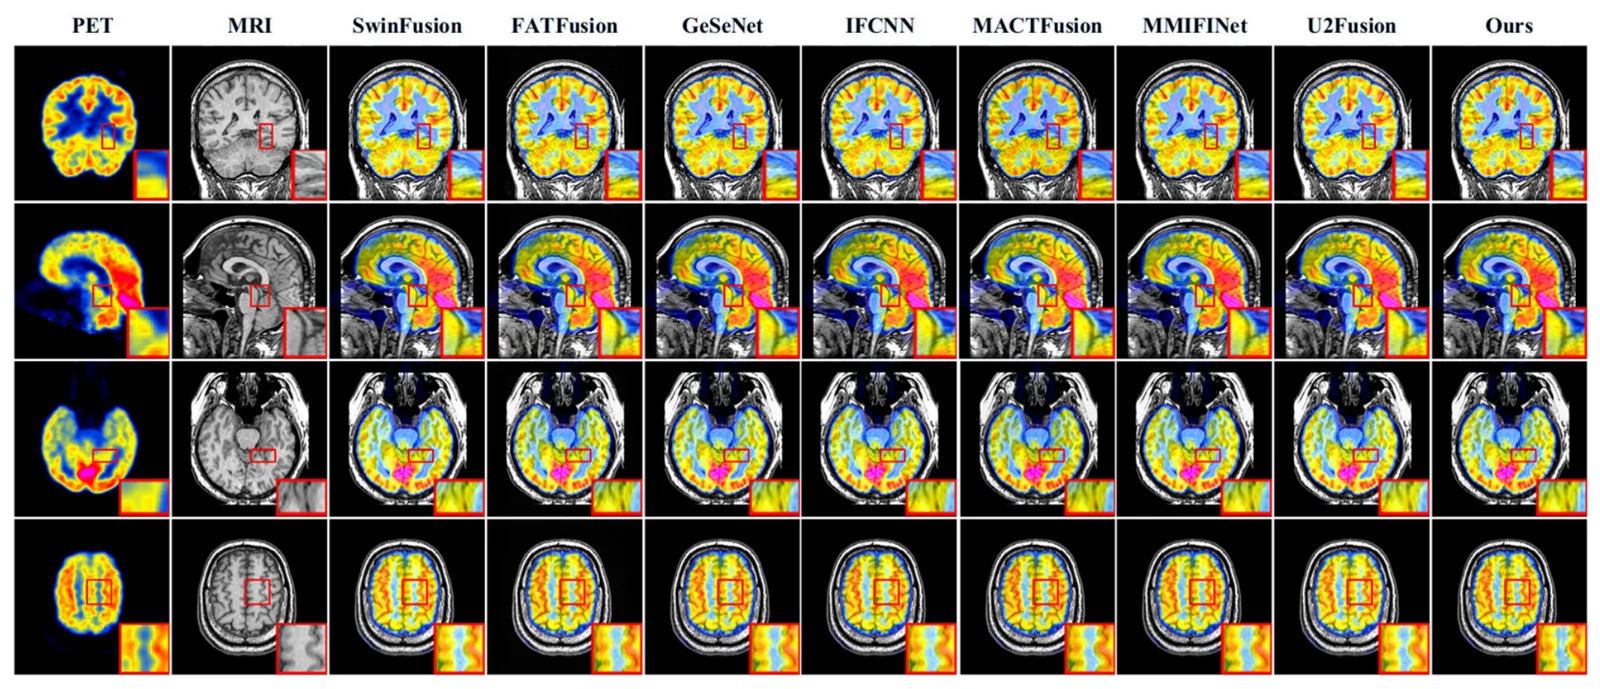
Qualitative comparison of fusion results on the PET–MRI dataset. Visual comparisons highlight the integration of functional metabolic information (from PET) with high-resolution anatomical structures (from MRI). The proposed MSFusion effectively retains the intensity and spatial localization of metabolic hotspots while maintaining clear anatomical contours, outperforming baselines that exhibit either color distortion, structural blurring, or loss of functional details. The red box indicates local zoomed-in details.
